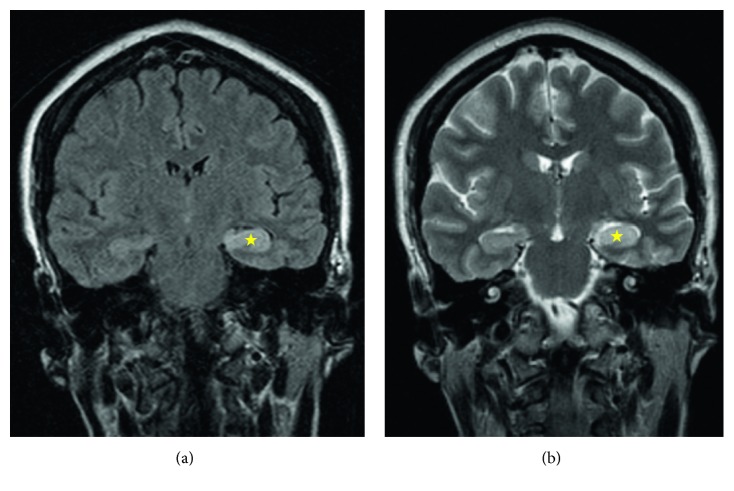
MRI brain with contrast on admission showing a hyperintense left hippocampus with mild swelling and contrast enhancement on (a) FLAIR sequence coronal section and (b) T2-weighted coronal section.
